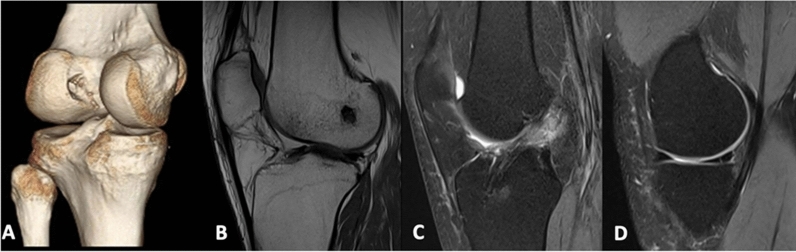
Example clinical case of femoral tunnel malposition. A 34-year-old patient, four years after ACLR, presented with 1 year of instability and intermittent pain, without a new traumatic event. Physical examination revealed positive pivot shift but negative Lachman and anterior drawer tests; medial meniscal tests were positive. A. CT 3D reconstruction demonstrated femoral tunnel malposition. B. T1 sagittal MRI confirmed tunnel malposition. C. Proton Density Fat-Suppressed (PD-FS) sagittal MRI showed a markedly thinned graft. D. PD-FS sagittal MRI revealed a medial meniscal tear
